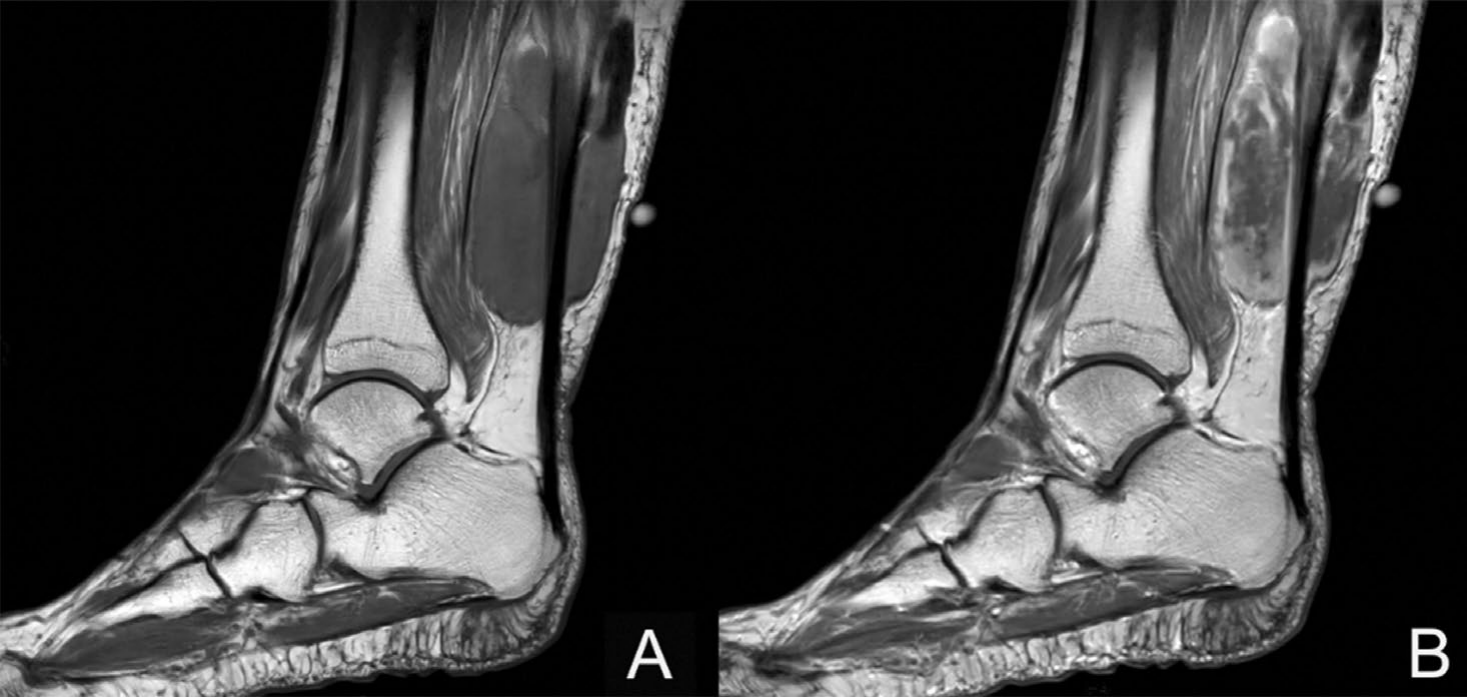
MRI showing a deep intramuscular mass related to the Achilles tendon. The tumor was hypointense in T1 sequence (A), with heterogeneous enhancing after contrast administration (B).
